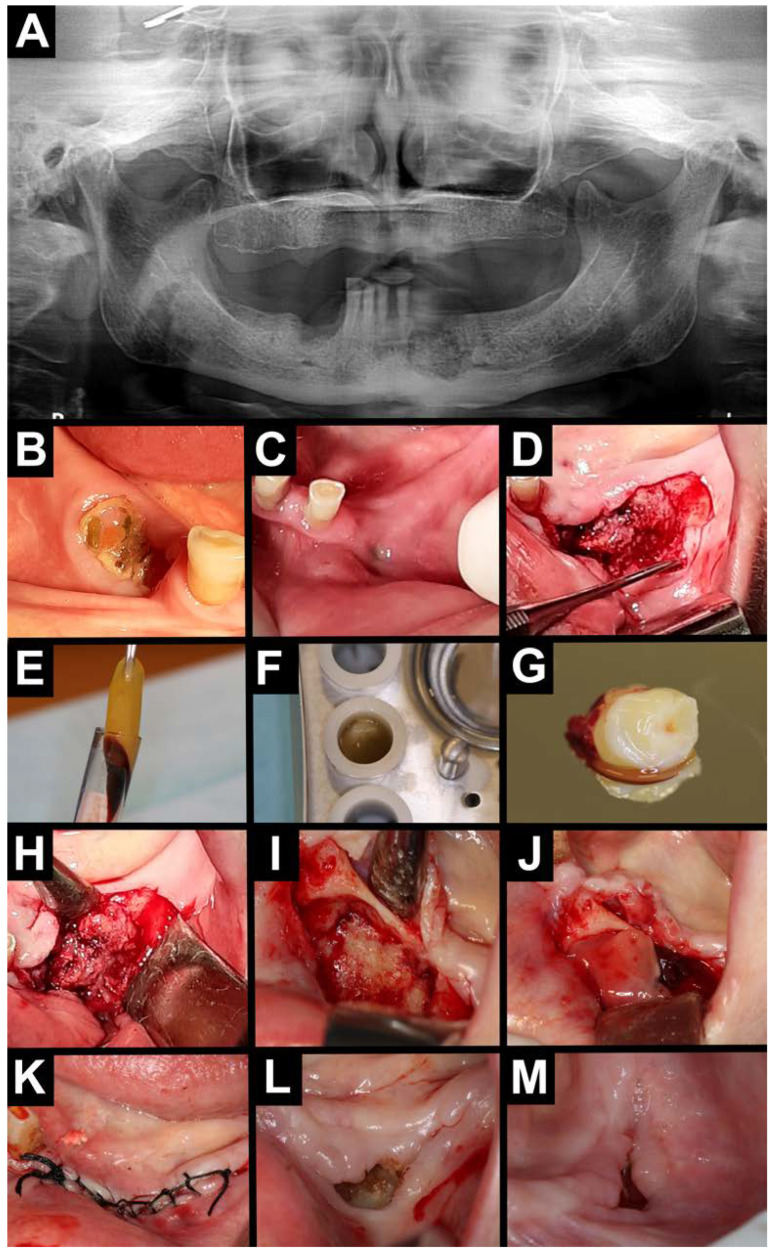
Treatment with A-PRF. (A) Orthopantomography—area with osteolysis and osteosclerosis on the left and right sides of the mandible. Intraoral photography (B–D). (B) Exposed necrotic bone on the right side of the mandible. (C) Intraoral purulent fistula on the left side of the mandible. (D) Visible necrotic bone after mucoperiosteal flap elevation on the left side of the mandible. A-PRF preparation (E–G). (E) A-PRF clot. (F) A-PRF in a PRF box. (G) A-PRF plug. Intraoral photographs (H–M). (H,I) Necrotic tissue was removed, and the bone was bled. (J) A-PRF plug placed intra-wound. (K) Wound was sutured. (L) Healing after 2 weeks. (M) Healing after 6 weeks.
